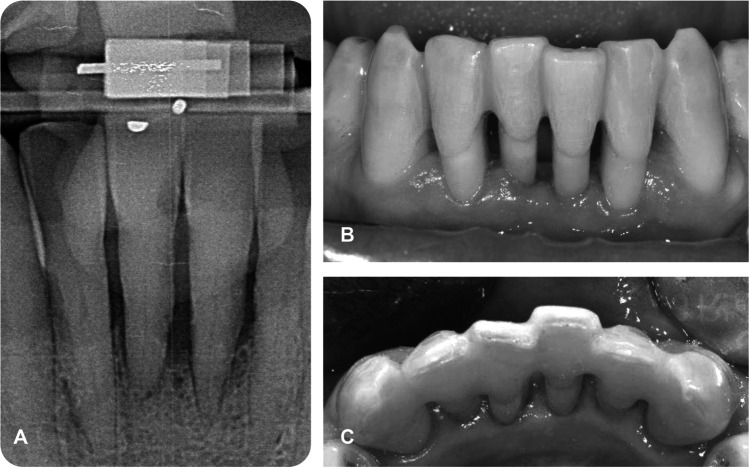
Standardized radiograph (A) of the mandibular front of a study participant prior to periodontal therapy (baseline) and clinical situation (B, C) 12 months after full-mouth disinfection and splinting of teeth 33 to 43 (T2)
